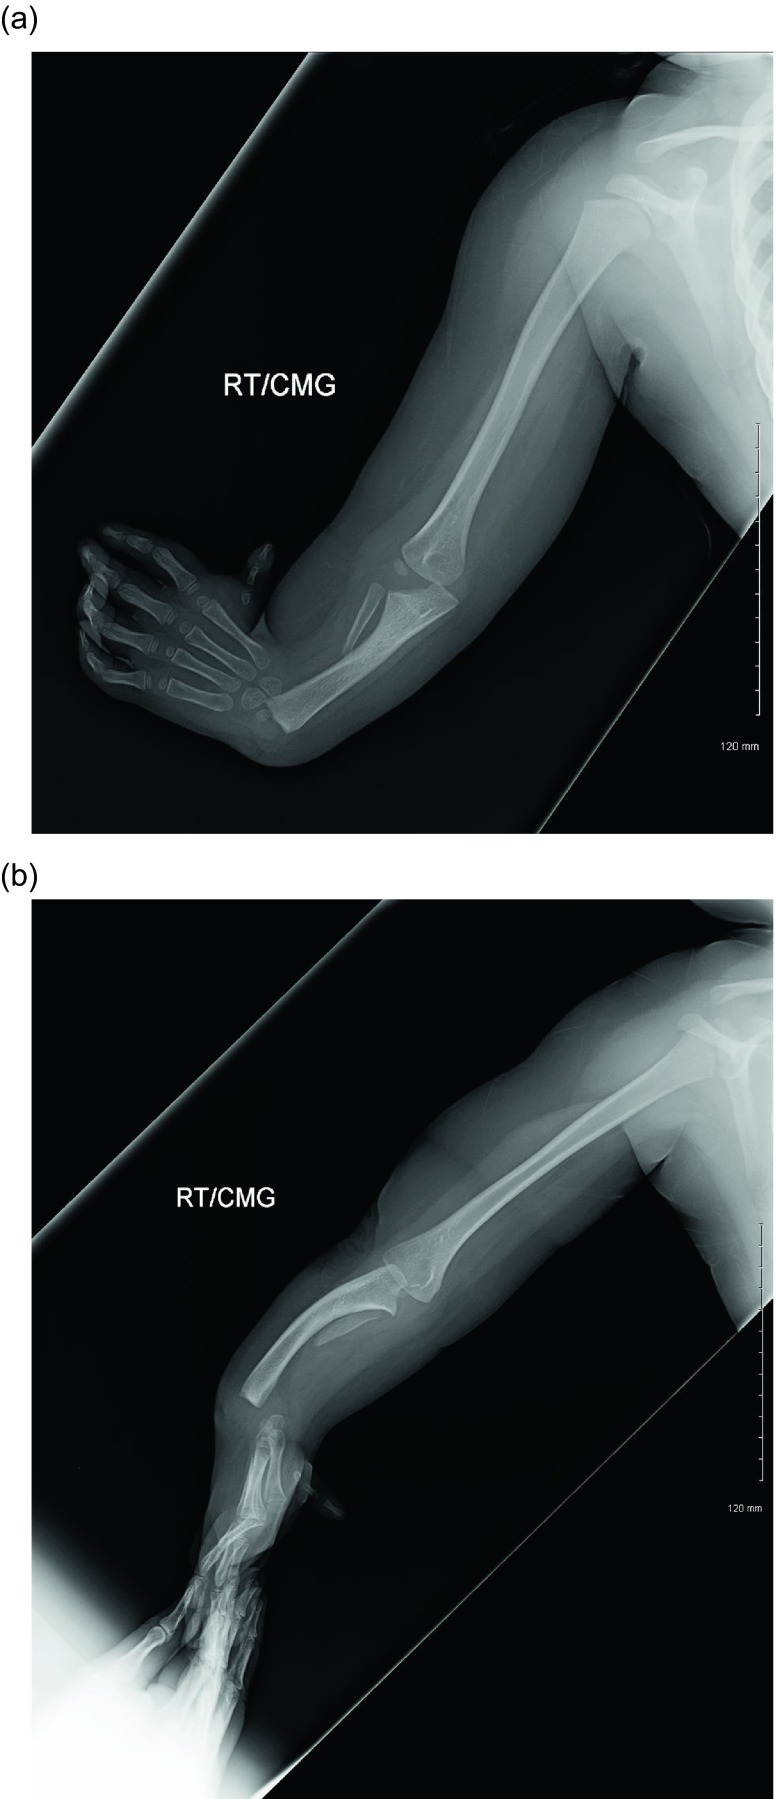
AP and lateral radiographs of left hand of a four-year-old boy with radial club hand before surgery.
